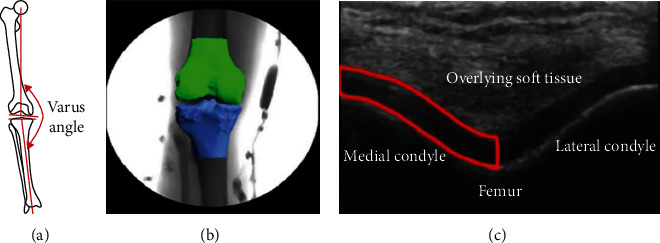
The morphological parameters of a knee joint. (a) Knee varus angle [23]. (b) Dynamical knee joint space [62]. (c) Ultrasonographic image of the femoral articular cartilage with the medial compartment cross-sectional area [64].
